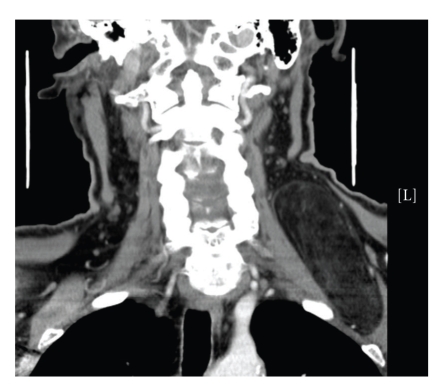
Coronal CT scan of the neck showing a left supraclavicular mass with internal septations.
